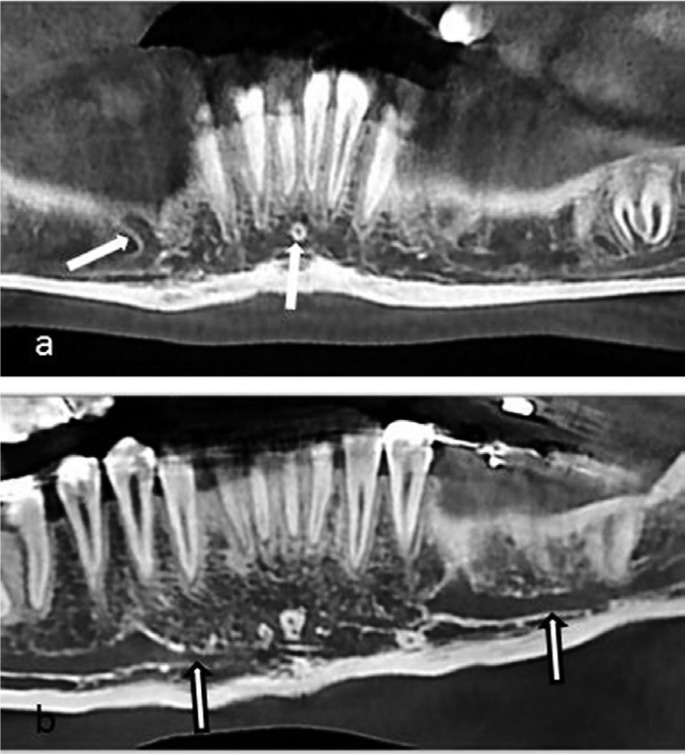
Cropped cone beam computed tomographic panoramic view of a 17-year-old boy with denoted structures; anterior looping of the mental nerve (right side) and lingual foramen (a), and incisive canal (right side) and mandibular canal (left side) of a 16-year old a girl (b).
